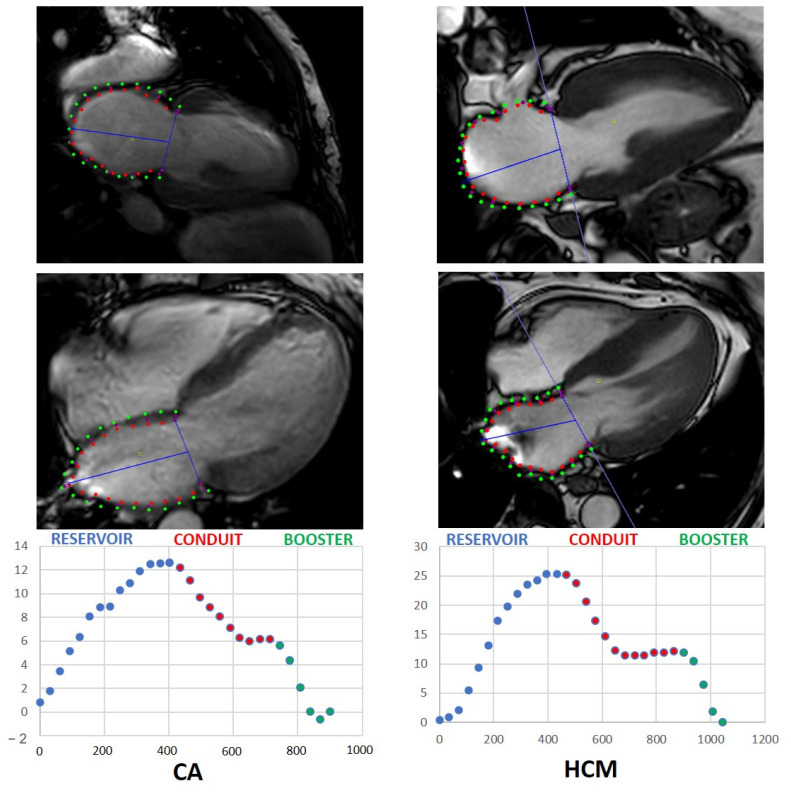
Left atrial strain measurements in patients with CA and HCM. (Upper row): longitudinal two-chamber view; (Lower row): left atrial longitudinal strain-to-time curve. Red dots in MRI images represent endocardial contours; green dots represent epicardial contours. CA: cardiac amyloidosis; HCM: hypertrophic cardiomyopathy.
